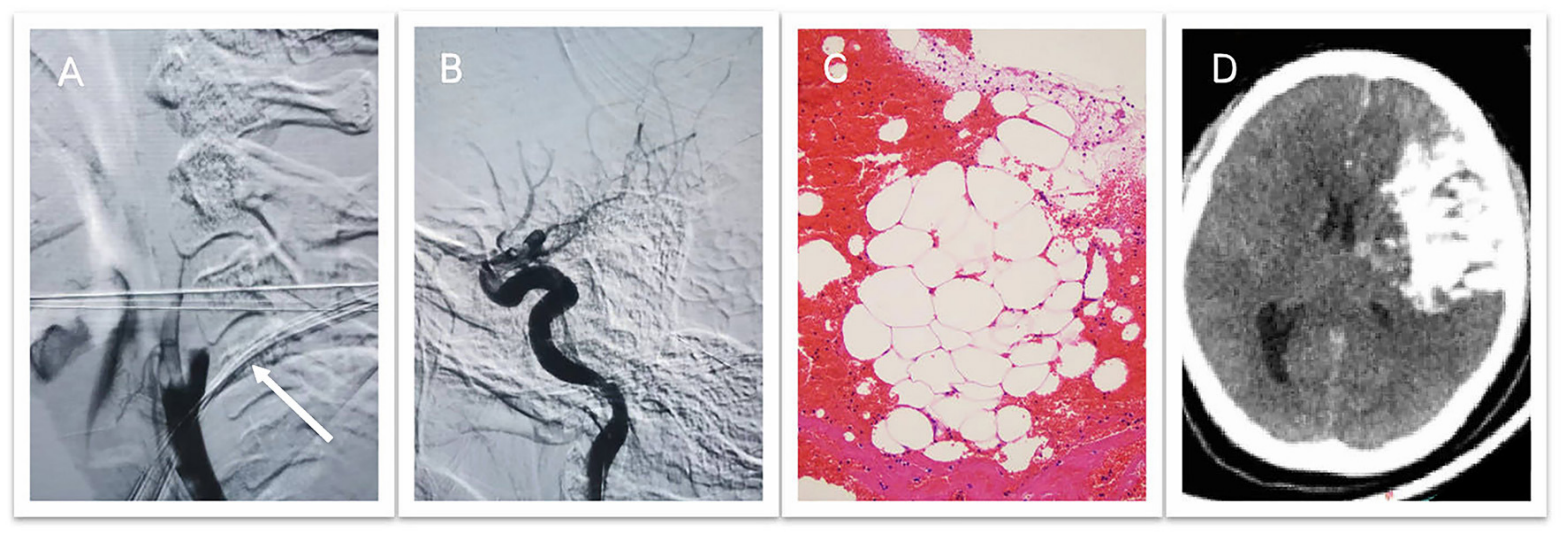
(A) Digital subtraction angiography confirms occlusion of the left internal and external carotid arteries. (B) Mechanical thrombectomy fails to achieve complete recanalization. (C) Pathological examination of the emboli confirms the presence of adipocytes. (D) Postoperative computed tomography demonstrates a large cerebral infarction with extravasation of the contrast agent in the left cerebral hemisphere and a slight rightward shift of the midline.
